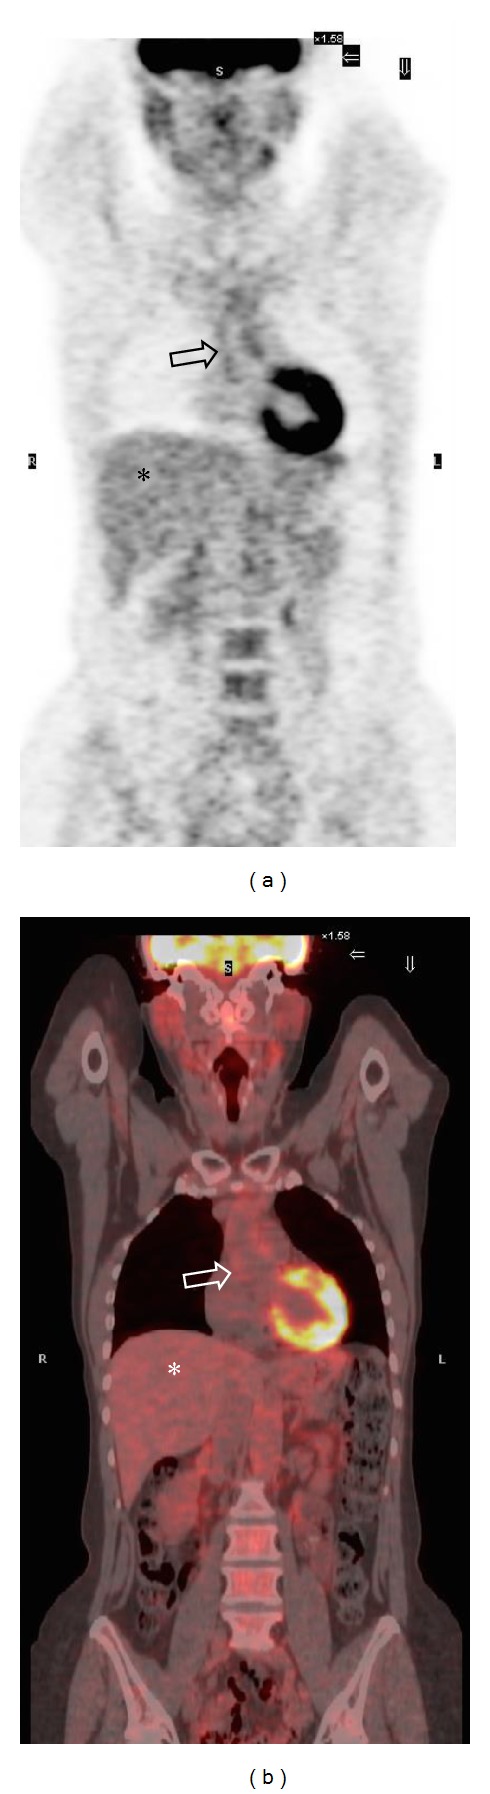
80-year-old male patient with clinical and PET-CT findings of giant cell arteritis. In this patient, coronal PET (a) and PET-CT (b) images demonstrate 18F FDG uptake of the walls of the ascending thoracic aorta (void arrows). The tracer uptake is similar to that of the liver parenchyma (asterisk), corresponding to grade 2 (significant vascular inflammation) according to the visual grading score proposed by Meller et al.
